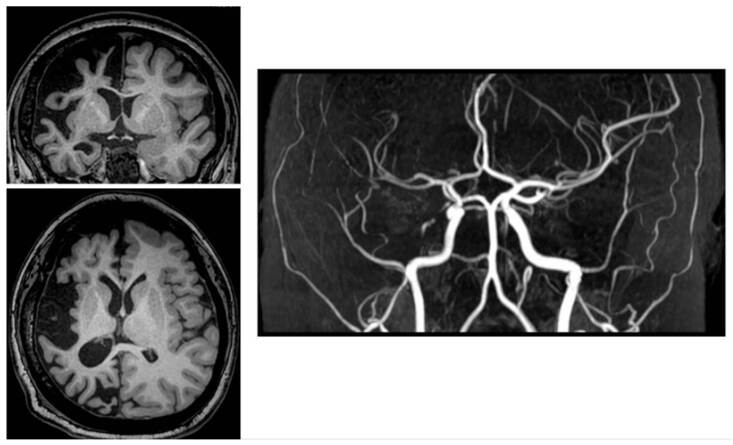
Cerebral magnetic resonance imaging and angiography. Diploe thickening over the right skull convexity, with asymmetric atrophy of the cerebral hemispheres, significantly greater on the right side. It involved all lobes and the midbrain due to hypoplasia of the right cerebral peduncle. Additionally, right hemispheric arteries appear smaller compared to their contralateral counterparts.
